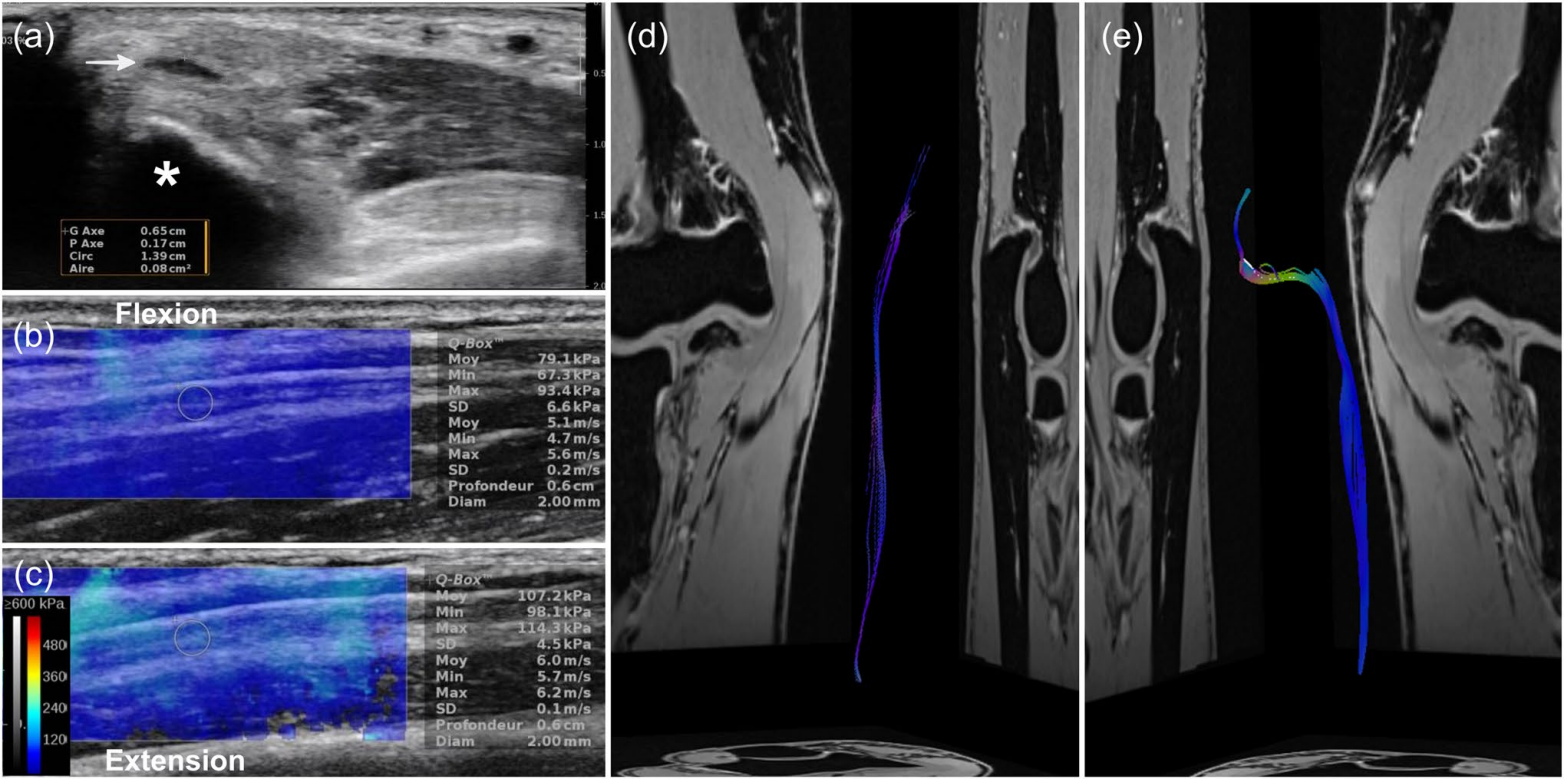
Conventional ultrasound and SWE, and DTI with fiber tractography. a Axial/transverse B-mode ultrasound image of the left ulnar nerve with CSA measurement at the compression site (0.08 cm^2^, white arrow). The ulnar nerve is located anterior to the medial epicondyle (asterisk). b SWE image and measurement of the left ulnar nerve in complete elbow flexion (mean, 79.1 kPa; SD, 6.6 kPa). c SWE image and measurement of the left ulnar nerve in complete elbow extension (mean, 107.2 kPa; SD, 4.5 kPa). DTI fiber tractography reconstructions of the right and left ulnar nerves. d Right elbow showing the normal pathway of the right ulnar nerve located posterior to the medial epicondyle within the cubital tunnel. e Abnormal “S-shape” pathway of the left ulnar nerve running anterior to the medial epicondyle outside the cubital tunnel
